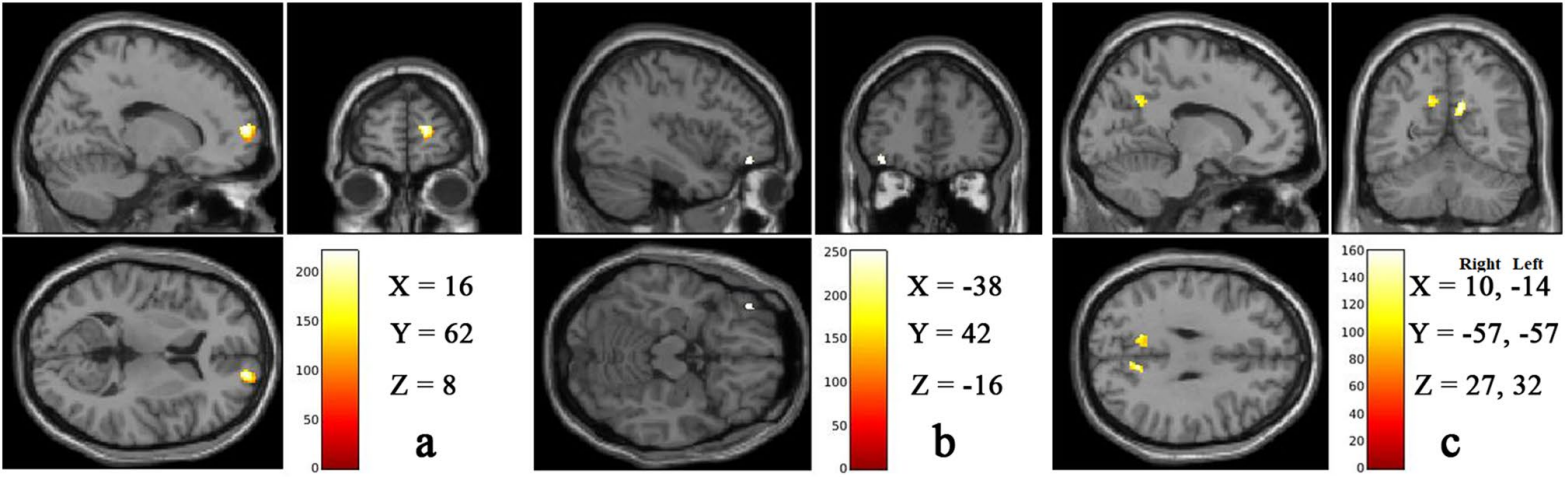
Representative structrual MRI images, showing significant difference (*p* < 0.05 FWE TFCE corrected) in (a) right superior frontal gyrus, (b) inferior orbitofrontal gyrus and (c) bilateral precuneus in RM practitioners than NM. Color bar represents TFCE-value. These representative structural MRI images were compiled and MNI coordinates were inserted into this compiled image using Adobe Photoshop version CS3 (https://www.adobe.com/in/).
